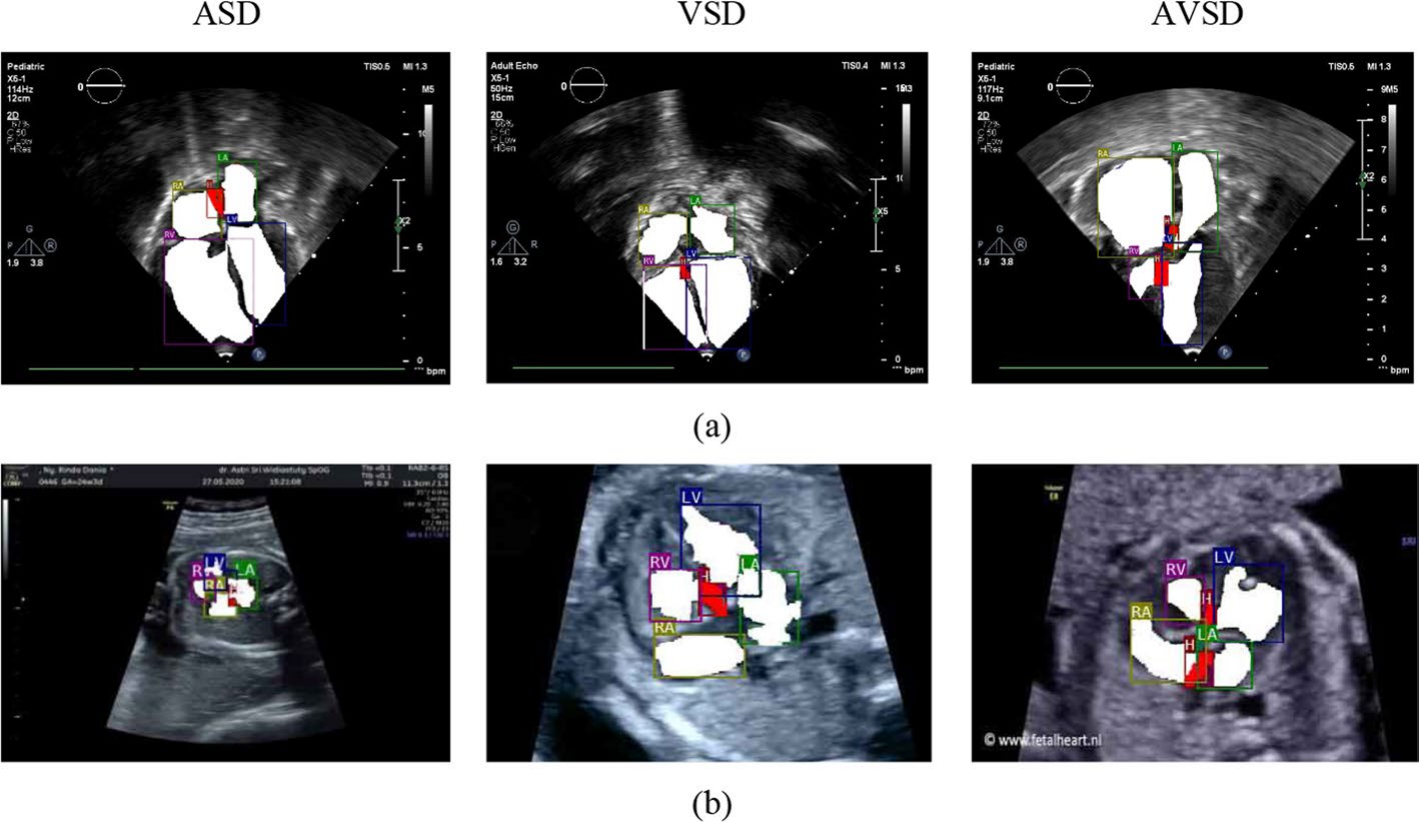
Example of the echocardiogram annotation process by experts to develop an AI model that can detect cardiac septal defects prenatally and postnatally. The cardiac chamber region is marked with white (right atrium [RA], left atrium [LA], right ventricle [RV], and left ventricle [LV]), whereas the defect area is marked with red. a Echocardiogram annotation of cardiac septal defect postnatally. b Echocardiogram annotation of cardiac septal defect prenatally
